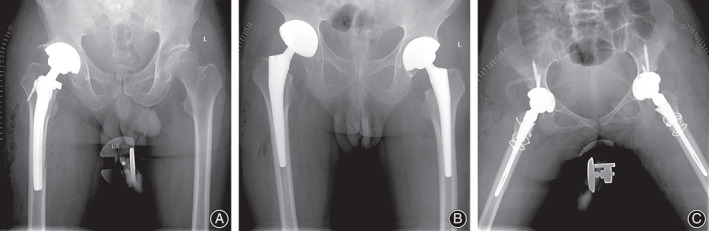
(A) A 65‐year‐old male underwent THA with a ribbed stem because of hip dysplasia and was found to have sustained an IPFF at the distal side of the right femur the day after surgery. No reoperation was performed because the fracture was regarded as stable. The patient was asked to delay weight‐bearing and received intensive care. The fracture was found to be healed at a subsequent follow‐up. (B) A 62‐year‐old male underwent one‐stage bilateral THA with a Corail stem because of hip fusion resulting from ankylosing spondylitis. Postoperative AP radiograph showed that the femoral stems did not match the medullary cavities and that a fracture of the distal femur was present on the right side, which had not been detected intraoperatively. The fracture healed 3 months postoperatively, and the patient had a good prognosis without reoperation. (C) A 25‐year‐old female underwent one‐stage bilateral THA with S‐ROM modular stems. Subtrochanteric shortening osteotomies were performed due to high hip dislocations. Postoperative radiograph showed a fracture line of the distal femur on the right side. The prophylactic cerclage wires were bound, reoperation was avoided, and bone union was observed at the 6‐month follow‐up
